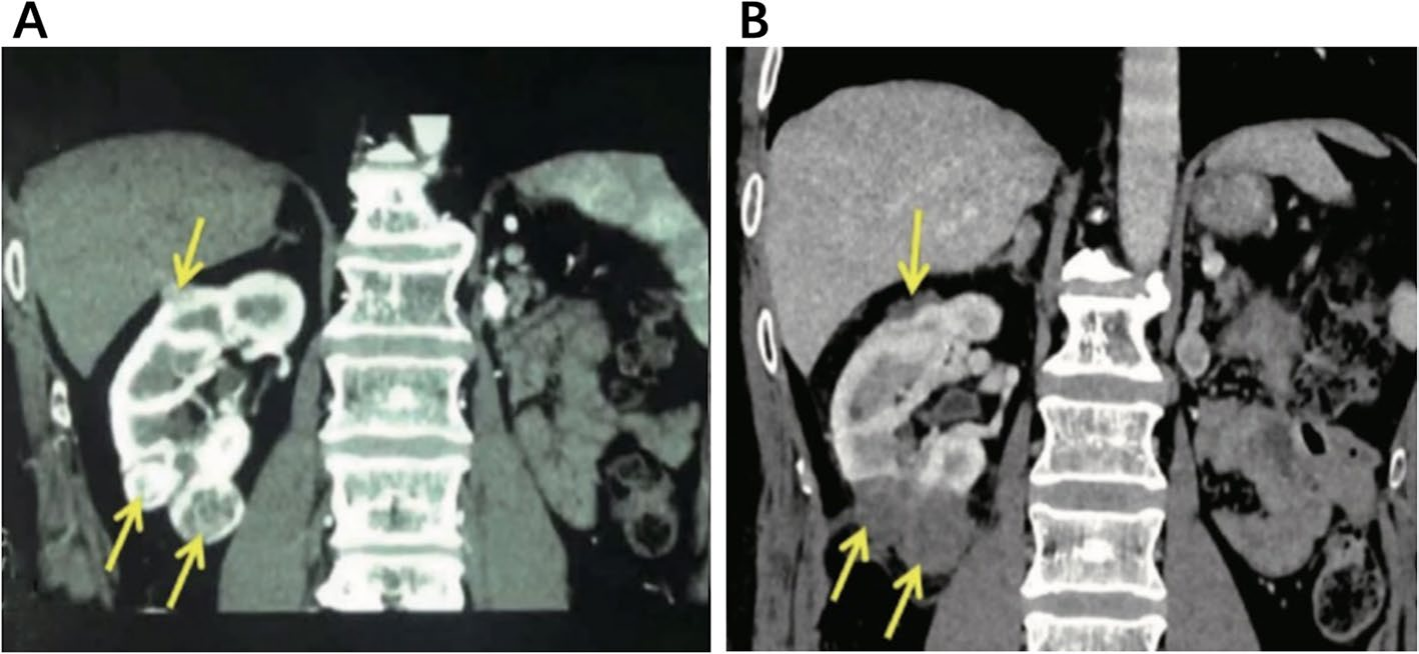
Before and after cryoablation in patients with renal cancer. A Preoperative contrast enhanced CT showed nonuniform enhancement of 3 tumors in the upper and lower poles of the right kidney (indicated by arrows). B Contrast CT showed no enhancement 1 week after cryoablation (shown by the arrows), indicating complete ablation of tumors
